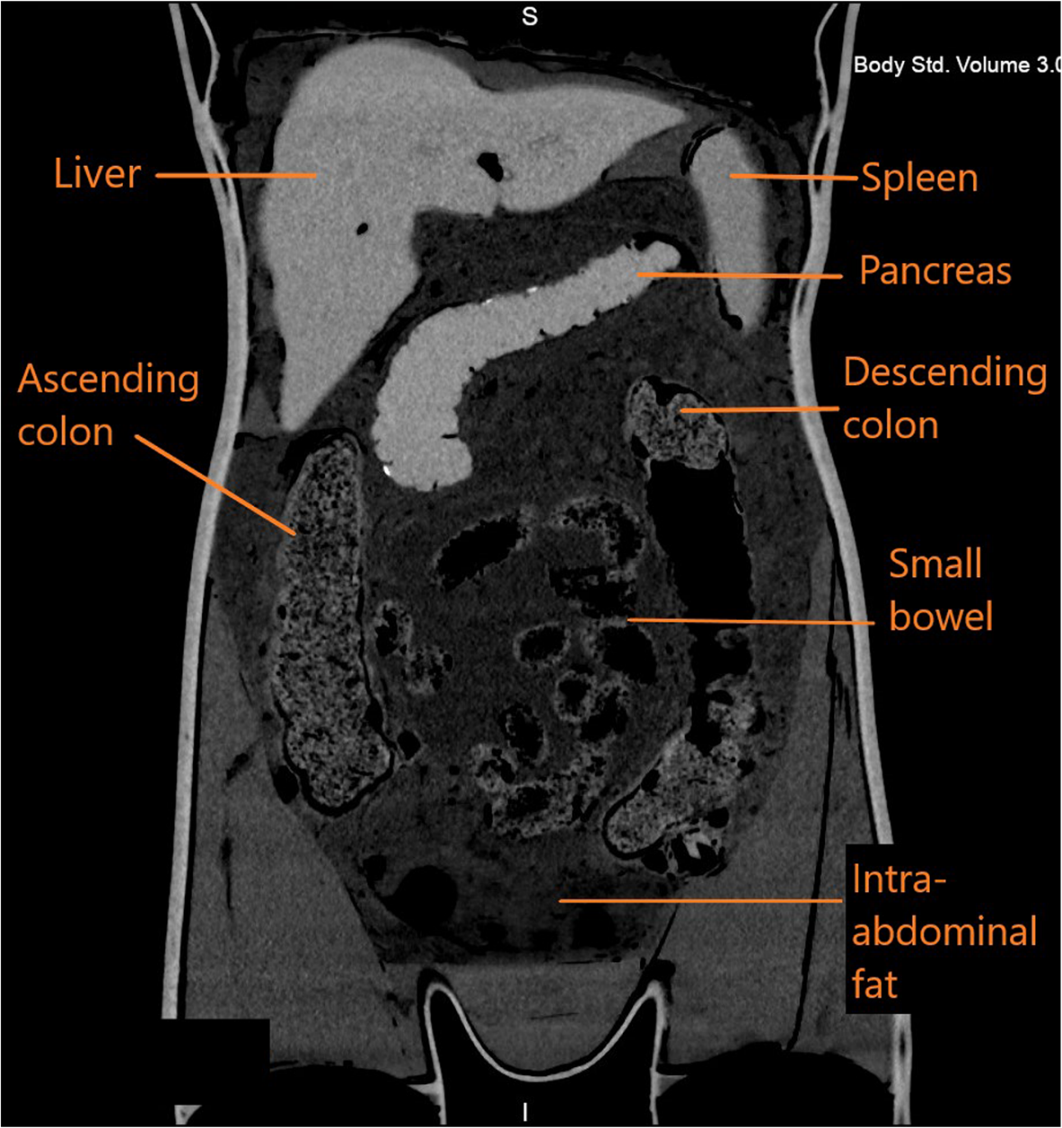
CT coronal view of CASMER demonstrates the positioned organs and surrounding intra-abdominal fat as labelled
